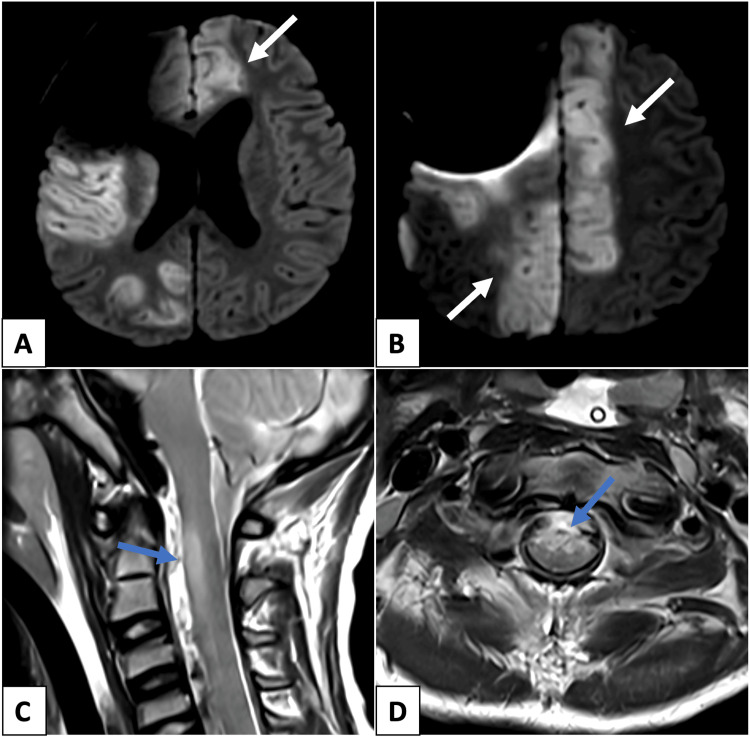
Magnetic resonance imaging of the brain at follow-up Axial brain diffusion-weighted imaging (A, B) shows extensive bilateral cortical ischemia involving the parasagittal regions (white arrow), accompanied by ventricular enlargement in (A). Sagittal (C) and axial (D) T2-weighted spine imaging reveal additional anterior cervical spinal cord ischemia (blue arrow).
